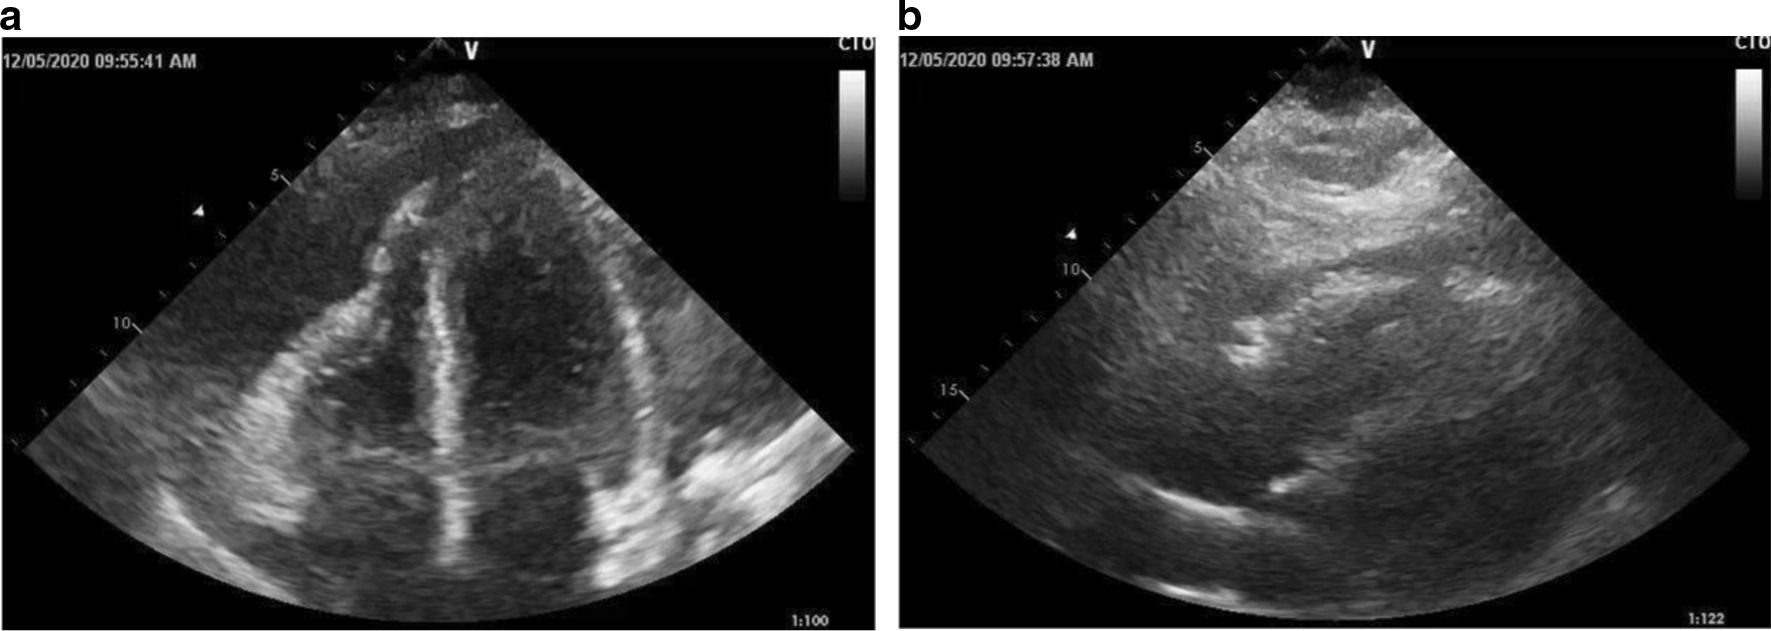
Repeat transthoracic echocardiogram with smaller pericardial effusion. a parasternal window, b subcostal window
